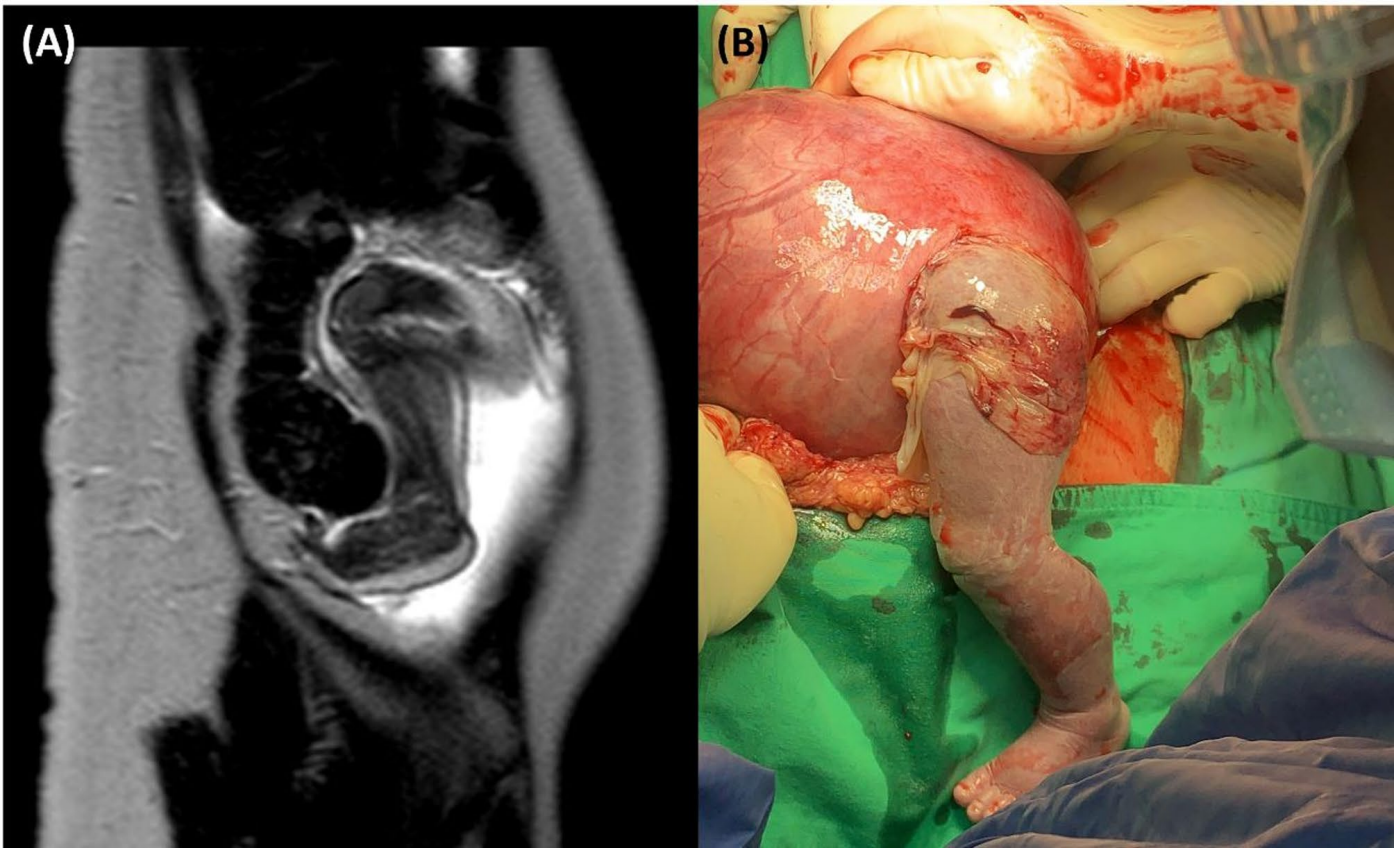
MRI and intraoperative images of uterine rupture with protruding fetal leg. A MRI image showing uterine rupture at the right interstitial region with the fetal leg protruding through the uterine wall (red arrow). B Intraoperative view confirming uterine rupture at the previous surgery site with the fetal leg protruding through the uterine defect (white arrow)
